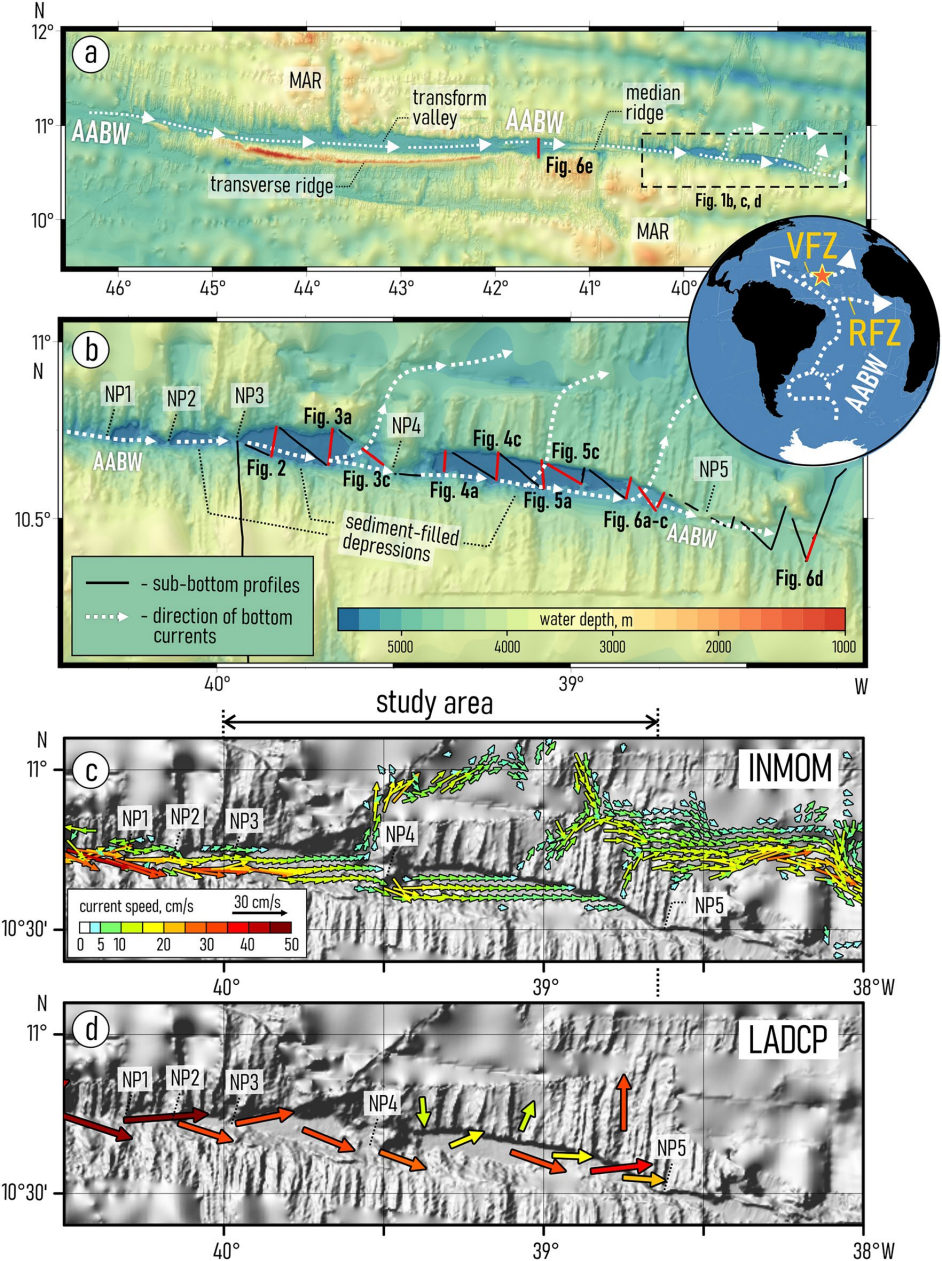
(a) The general bathymetry of the Vema Fracture Zone^36^ with the general directions of the AABW current^19^; dashed square marks the location of the study area, red lines mark sub-bottom profiles presented in this work. (b) Bathymetric scheme of the study area^36^ with location of the sub-bottom profiles and directions of the AABW flow, red lines mark the sub-bottom profiles presented in this work; (c) Results of numerical modeling of bottom current velocities in the study area using the Institute of Numerical Mathematics Ocean Model (INMOM); (d) Results of direct measurements of bottom current velocities using a Lowered Acoustic Doppler Current Profiler (LADCP). The inset at the top right shows the general directions of the AABW propagation from the SW to the NE Atlantic^19,36^, star marks the location of the study area. *MAR*—Mid-Atlantic Ridge, *VFZ*—Vema Fracture Zone, *RFZ*—Romanche Fracture Zone; *NP1-5*—narrow passages described in the text. Bathymetric schemes from (a–d) are based on the GEBCO_2022 data set (https://www.gebco.net/data_and_products/gridded_bathymetry_data/gebco_2022/).
